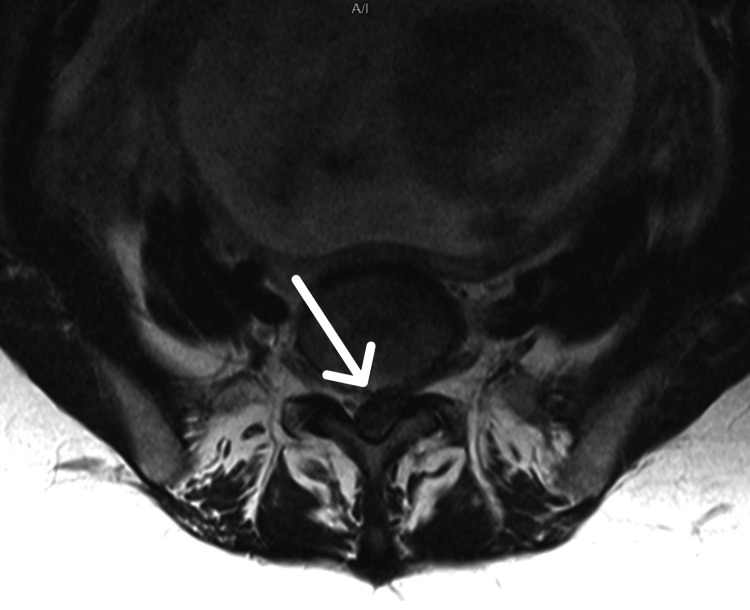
MRI Lumbar Spine: T2-weighted axial view at L5/S1 level demonstrating almost complete filling of the spinal canal with extruded disc
